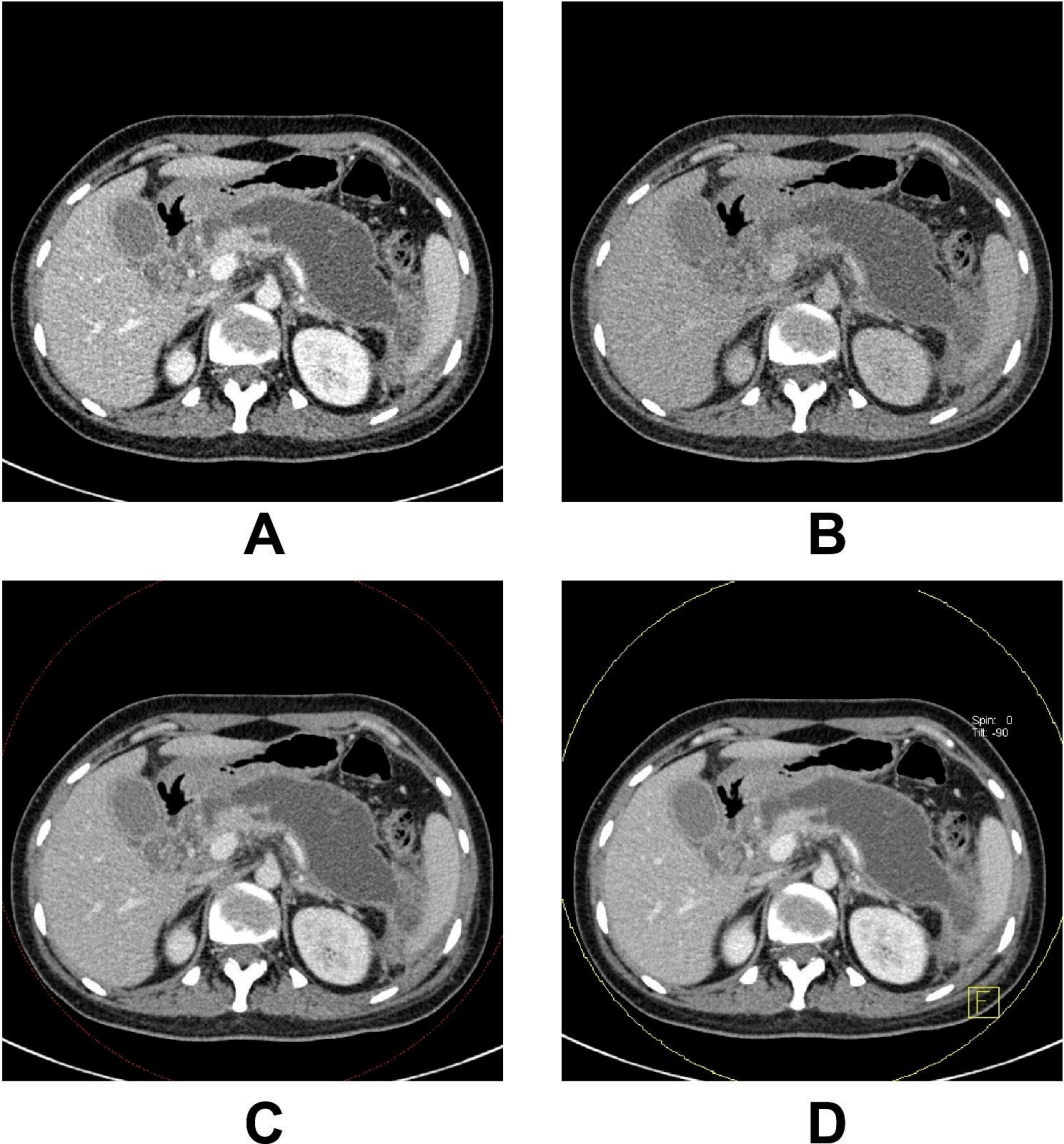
The images of 100 kV (A), Sn140 kV (B), weighted-average 120 kV (C) and the optimal single-energy level for CNR (D) acquired from contrast-enhanced dual-source dual-energy CT in portal venous phase of acute necrotising pancreatitis
